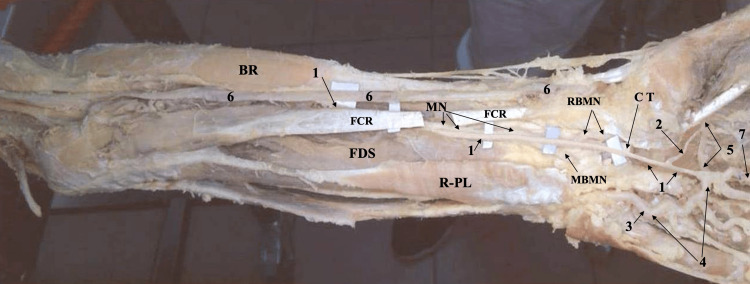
Gross image of the left forearm and hand showing the persistent median artery, bifid median nerve, and reversed palmaris longus 1. PMA, 2. A branch connecting the PMA to the DPA, 3. Ulnar artery, 4. Superficial palmar arch, 5. Deep palmar arch, 6. Radial artery, 7. The first common palmar metacarpal artery MN: Median nerve, RBMN: Radial branch of median nerve, MBMN: Medial branch of median nerve, BR: Brachioradialis muscle, FCR: Flexor carpi radialis muscle, FDS: Flexor digitorum superficialis muscle, R-PL: Reversed palmaris longus muscle, CT: Cut tendon belonging to FDS
